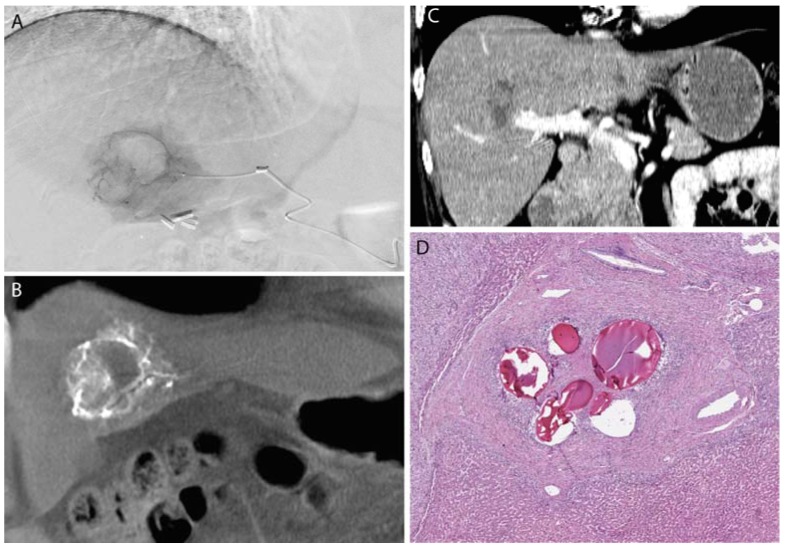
A leiomyosarcoma metastasis to the liver was embolized with 100–300 μm, doxorubicin-eluting microspheres to reduce tumor size and vascularity prior to surgical resection. Selective catheterization (A) of the tumor was performed, with proper positioning confirmed by cone-beam CT (B). Post-procedure CT (C) demonstrates no residual vascularity within the lesion. Following liver resection, microspheres were identified within the blood vessels in the tumor bed without any evidence of viable metastatic tumor (D).
